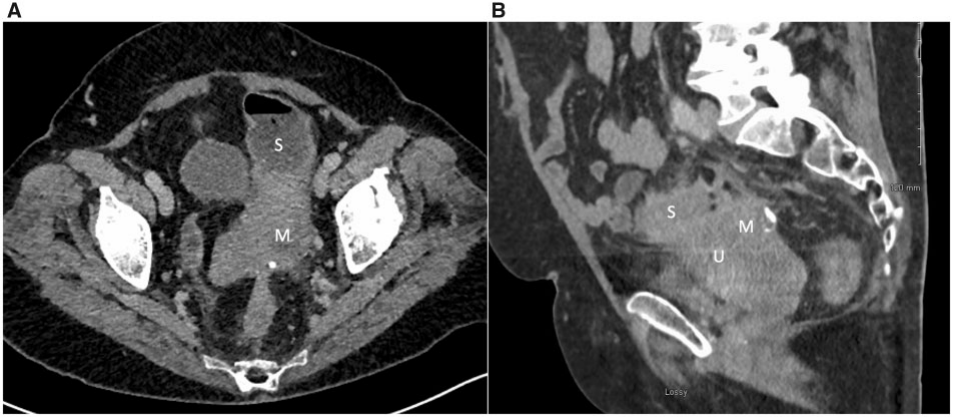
(A) Axial CT of the pelvis. A heterogenous mass is noted at the left side of the pelvis, with internal foci of calcification. Given the appearance of involvement of the sigmoid colon, this was initially felt to represent an inflammatory mass secondary to perforated sigmoid diverticulitis. (M—mass) (S—sigmoid colon). (B) Follow-up CT performed a month later demonstrates how the ill-defined mass (M) is directly involved with the fundus of the uterus (U). A loop of inflamed sigmoid colon (S) is visualized anterosuperior to the mass.
